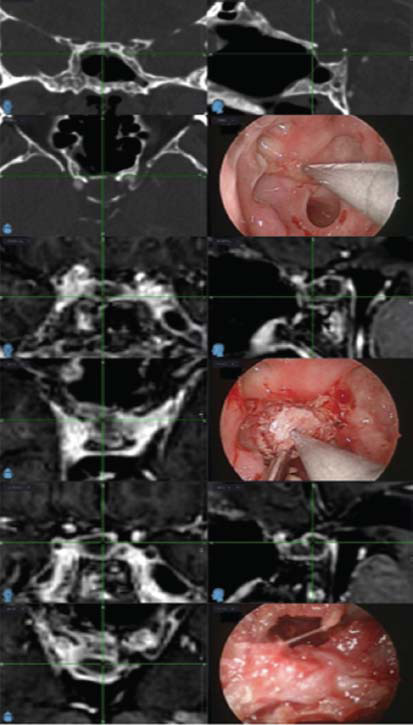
Screenshots are taken during the second ETSS procedure with guided MRI after abscess recurrence.
